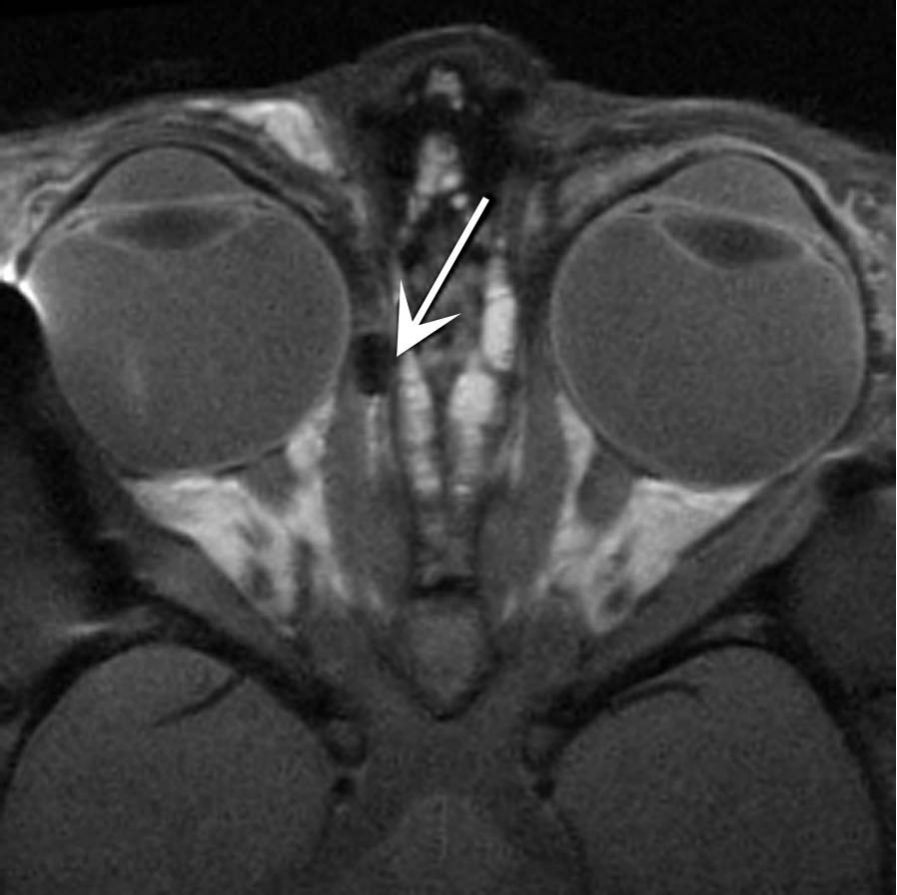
Magnetic resonance imaging (MRI) of M1 at 2 months showing the pellet in place on the medial rectus muscle (*arrow*) performed using a Siemens 3-T imager, *T*_1_-weighted fast spin-echo.
